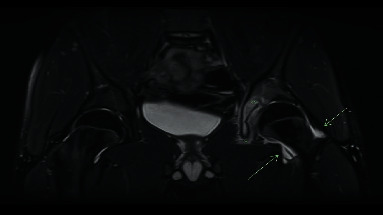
MRI T2 fat-saturated coronal image demonstrating acetabular and periacetabular soft tissue edema (*∗*) and a small joint effusion (arrow) in the left hip.
